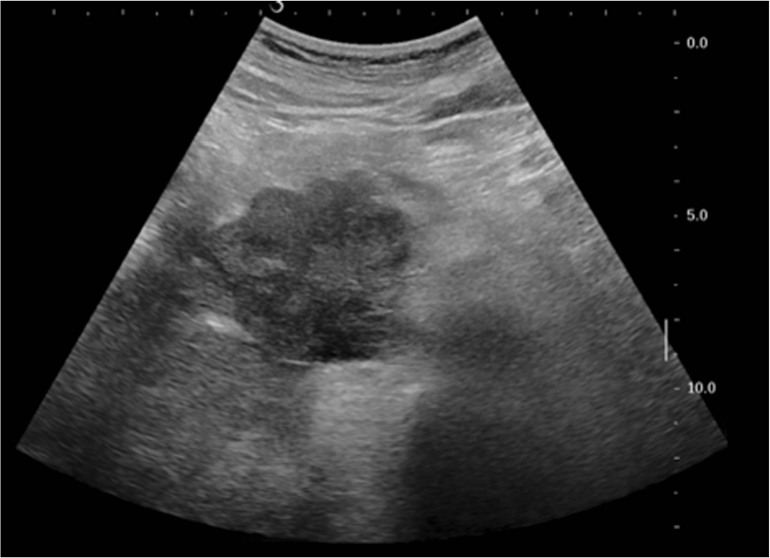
Ultrasound: An irregular, lobulated mass in the head of the pancreas
